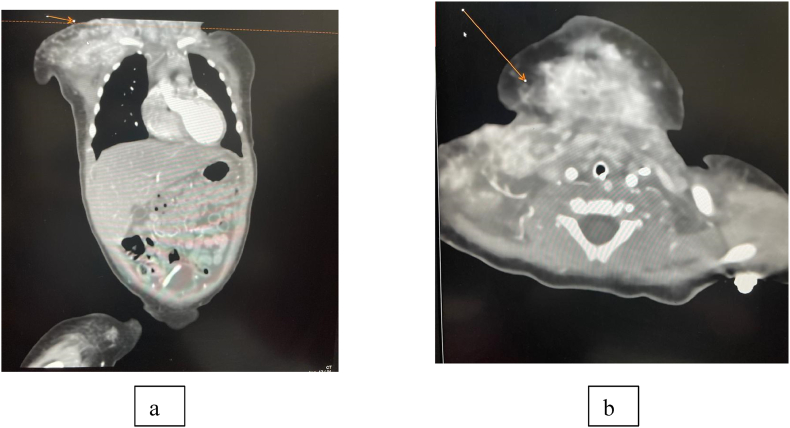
Post-contrast CT of the chest showing an avidly enhanced mass lesion seen in the right chest wall (a) extending to the visualized lower neck and shoulder region with multiple related enhancing vessels representing the feeding arteries and draining veins (b). The orange arrow on both images (a, b) marks the lesion site. There is incidentally noted right sided pneumothorax and left lower lobe patch of consolidation. . (For interpretation of the references to colour in this figure legend, the reader is referred to the Web version of this article.)
